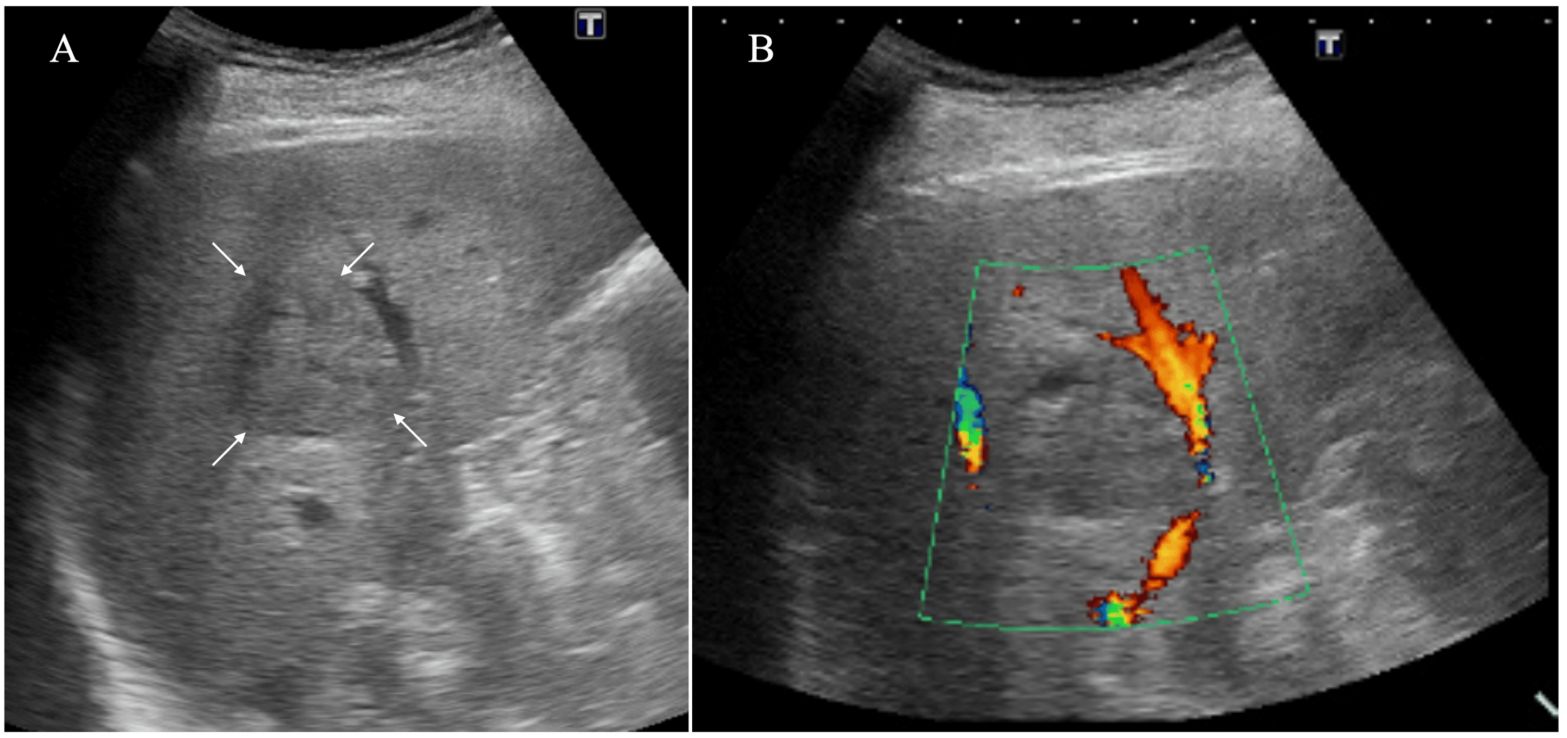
Ultrasound findings Ultrasound showed a round mass with enhanced posterior echoes (A, arrows), blood flow around the mass, and no blood inflow to the mass (B).
